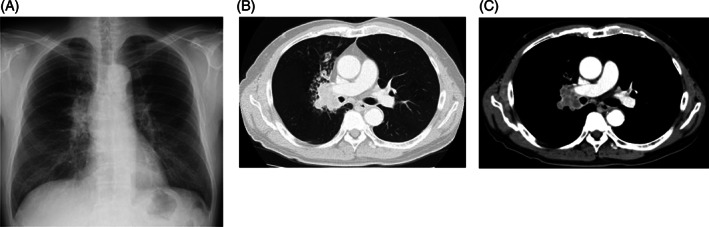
(A) Chest X‐ray and (B, C) contrast‐enhanced CT at the first admission. Chest X‐ray and contrast‐enhanced CT showed a tumor in the right upper lobe
